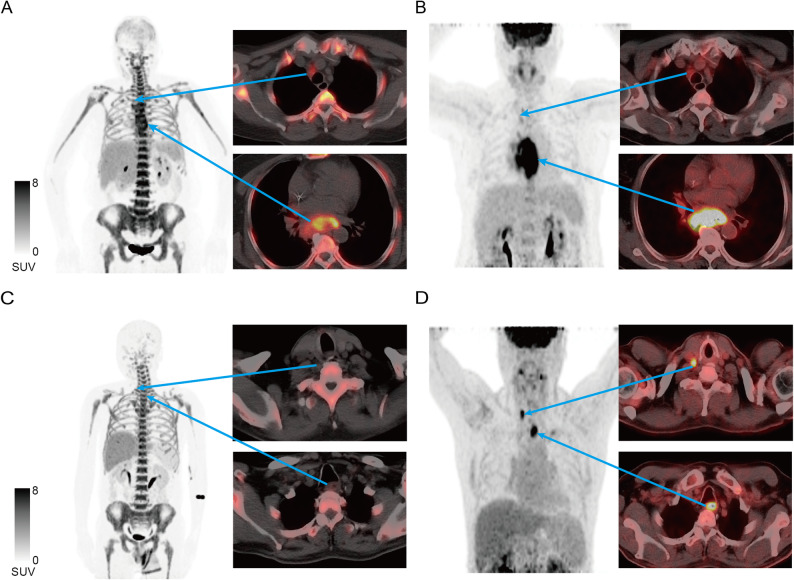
Representative ^18^F-FDG and ^18^F-FLT PET/CT images of two patients with locally advanced oesophageal squamous cell carcinoma (LA-ESCC). A, B: Patient 16 (poor prognosis): A: ^18^F-FLT PET/CT showing high uptake in metastatic lymph node and primary tumour; B: ^18^F-FDG PET/CT of the same patient showing high uptake in both lesions.C, D: Patient 4 (good prognosis): C: ^18^F-FLT PET/CT showing low uptake in both lesions; D: ^18^F-FDG PET/CT of the same patient showing high uptake in both lesions
